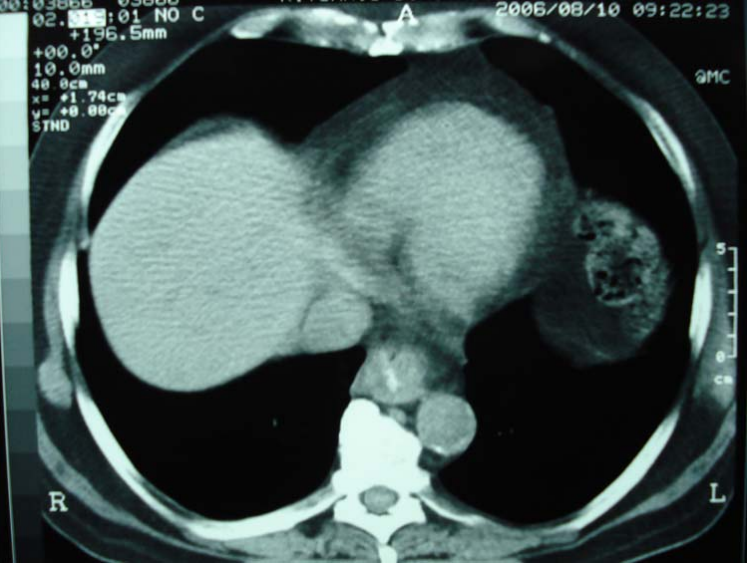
Post-chemotherapy CT scan showed a significant reduction of the tumor size.
